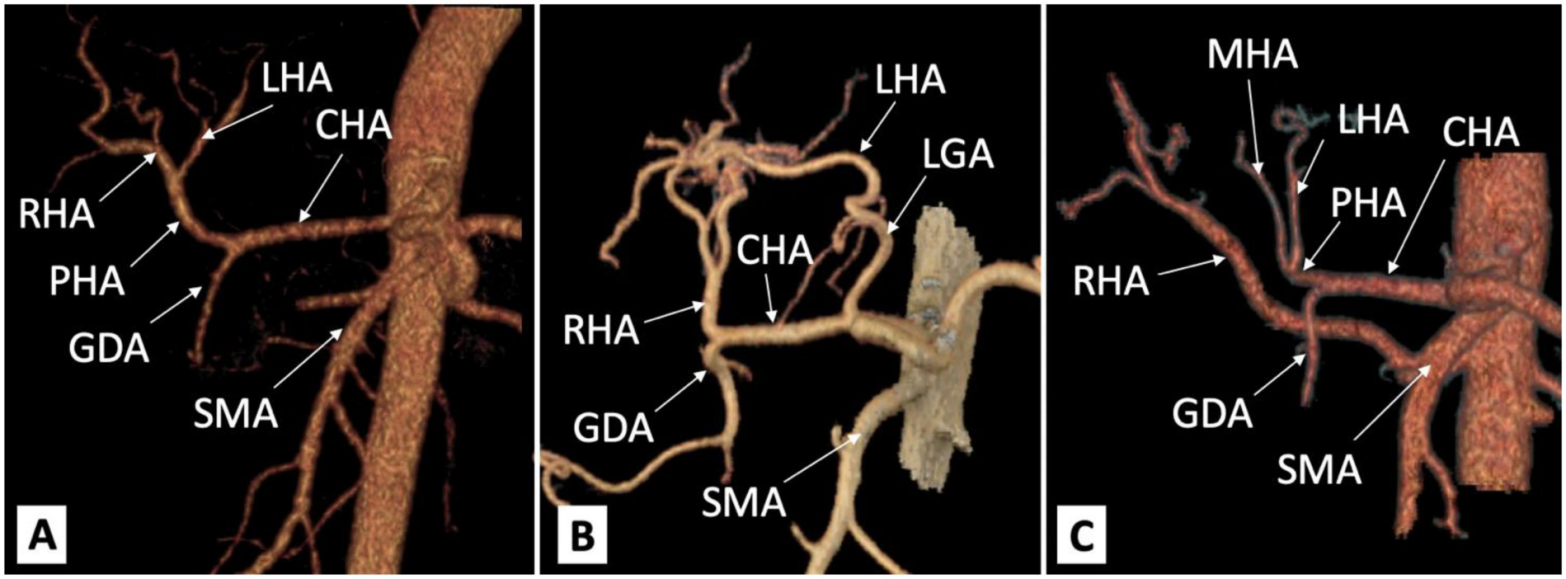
Common hepatic arterial variants on 3D computed tomography reconstruction: (A) Michel's type I (normal liver artery anatomy); (B) Michel's type II (LHA from LGA); (C) Michel's type III (RHA from SMA). CHA, common hepatic artery; GDA, gastroduodenal artery; LGA, left gastric artery; LHA, left hepatic artery; PHA, proper hepatic artery; RHA, right hepatic artery; SMA, superior mesenteric artery.
